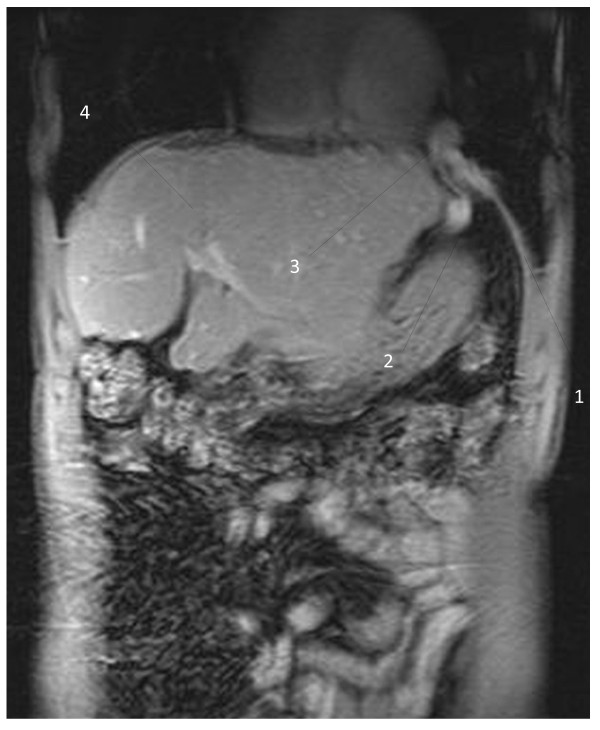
T1-weighted magnetic resonance image of the vascular diaphragmatic hernia (after contrast administration), coronal view. 1 - Left diaphragm muscle; 2 - abdominal part of the herniated vein; 3 - diaphragmatic defect corresponding to the base of the vascular loop; 4 - liver.
